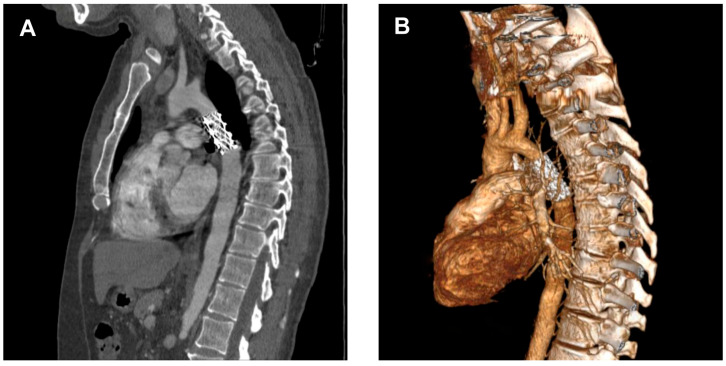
Six-month follow-up imaging after endovascular treatment of thoracic aortic atresia. (A) Sagittal reconstruction of contrast-enhanced computed tomography (CT) demonstrates patency of the thoracic aortic stent-graft with preserved alignment and no evidence of endoleak. (B) A three-dimensional volume-rendered CT image confirms the proper stent position and unobstructed aortic flow, with no signs of graft migration or stenosis.
